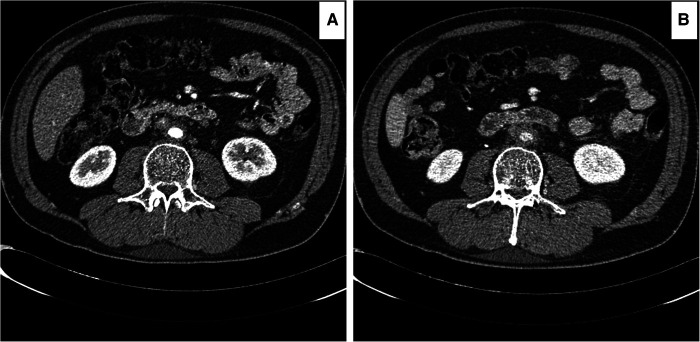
Computed tomography angiography (CTA) images showing worsening of the peri-aortic soft tissue after medical management. (A) RPF with less than 3 mm enhancements around the aorta and 21 mm aortic diameter. (B) after six months of steroid and mycophenolate, the enhancements increased to 5 mm, and the aortic diameter decreased to 18 mm. The patient had continuous abdominal and low back pain, not responding to the WHO one and two pain ladder medications.
